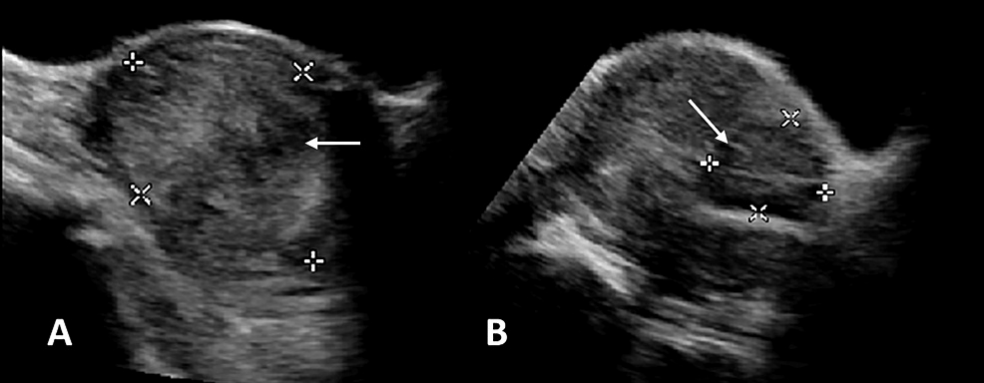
Ultrasound images of the uterine intramural (A) and subserosal (B) masses show well-defined boundaries and similar echogenicity.
